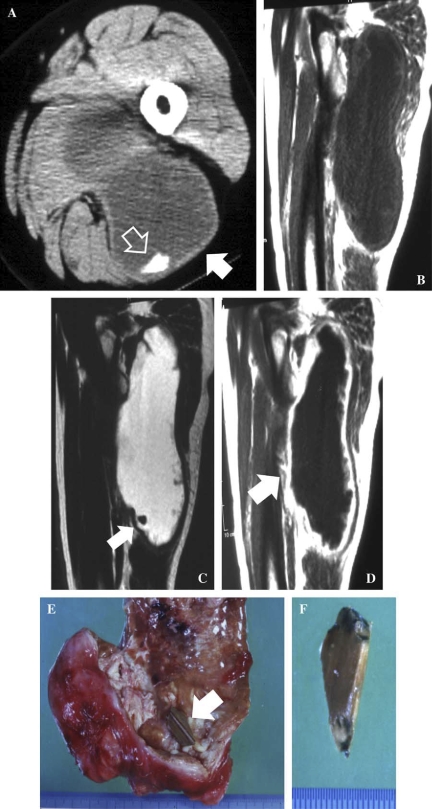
Fifty-five-year-old woman with foreign body granuloma of the left posterior thigh. A: Axial computed tomography (CT) of the left thigh. Huge mass in the hamstring muscle (solid arrow) with a small high-density fragment (open arrow) was observed. B and C: Sagittal T1- and T2-weighted images of the left posterior thigh. The mass had low intensities on T1- and high intensities on T2-weighted images to the muscles. The small fragment inside the mass showed low intensities to the muscles (arrow). D: The peripheral area of the lesion (arrow) was strongly enhanced after gadolinium injection. E: Cut surface of the lesion. The mass was filled with much fluid and granulation tissue. A foreign body (arrow) was seen inside the mass. F: A fragment of tile (3×1 cm) was removed at surgery.
